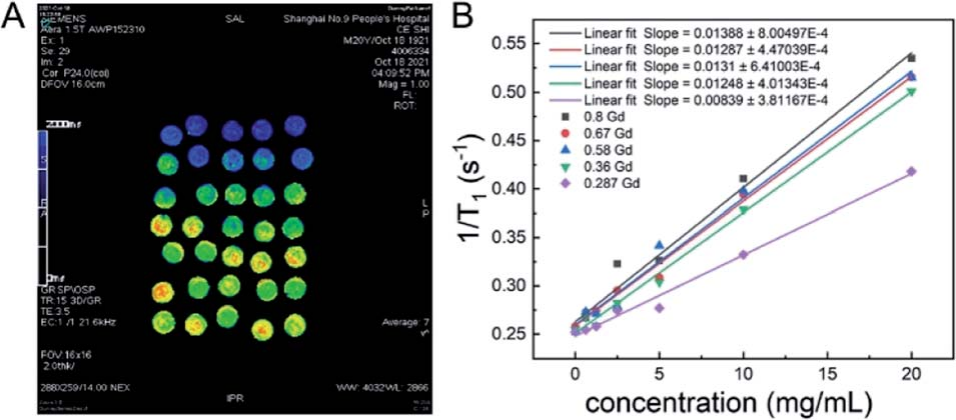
(A) *T*_1_ mapping images of MRI, and (B) the relationship between *T*_1_ relaxation rates and the sample concentration at different Gd concentrations.
